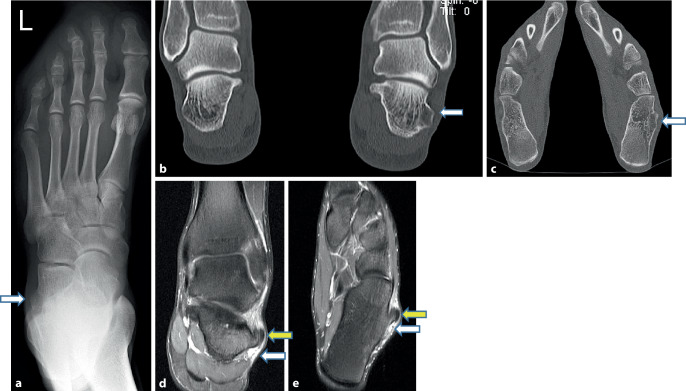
Röntgen‑, CT- und MRT-Befunde, a Das dorsoplantare Röntgenbild zeigt die hypertrophierte Trochlea peronaealis links (*Pfeil*), b, c koronarer und axialer CT-Schnitt zeigen im Seitenvergleich die erhebliche ossäre Hypertrophie der Trochlea peronaealis links mit 6–8 mm Differenz (*Pfeile*), d, e koronarer und axialer MRT-Schnitt zeigen die Luxation der M.-peronaeus-longus-Sehne nach kranial auf Trochlea und M.-peronaeus-brevis-Sehne reitend (*gelber Pfeil*) sowie das leere Fach unterhalb der Trochlea peronaealis (*weißer Pfeil*)
